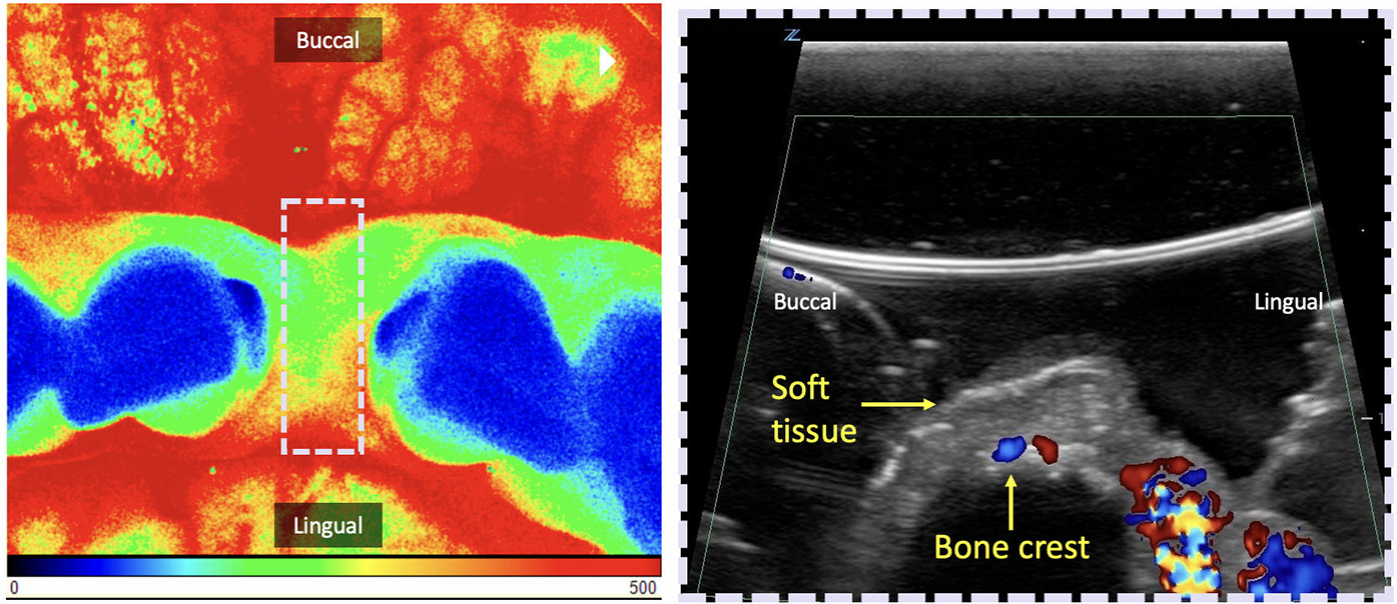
Demonstration of two perfusion imaging techniques of the edentulous crest. Both can be used in the occlusal view for pre-assessment of future implant placement in the anterior mandible. *Left:* Laser Speckle Contrast Imaging (LCSI). Here, blood flow is expressed through Laser Speckle Perfusion Units (LSPU) and depicted as false color images: blue-cyan (low perfusion), green-yellow (moderate perfusion), orange-red (high perfusion). The region of interest (ROI) delimited by the dashed white rectangle is the location of the ultrasound shown on the right. *Right:* Cross-sectional B-mode ultrasound image with color flow in velocity mode. Color velocity images display hues of blue to red, which correspond to the detected blood flow velocity. Changes in this velocity potentially serve as a quantitative indicator for the degree of inflammation. In this case, in both techniques, the presence of high tissue perfusion can be seen on the lingual surface and low to medium perfusion mid-occlusal.
